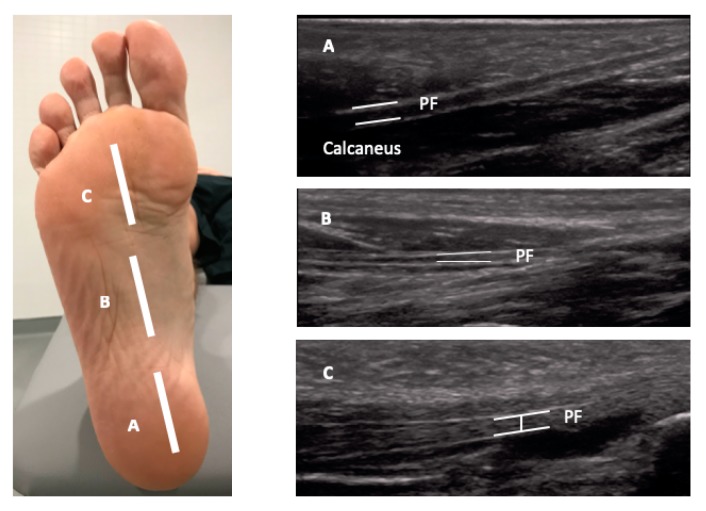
Ultrasound imaging thickness for the plantar fascia at the insertion, midfoot and forefoot locations in an individual with Achilles tendinopathy (AT). Abbreviations: PF, plantar fascia.
